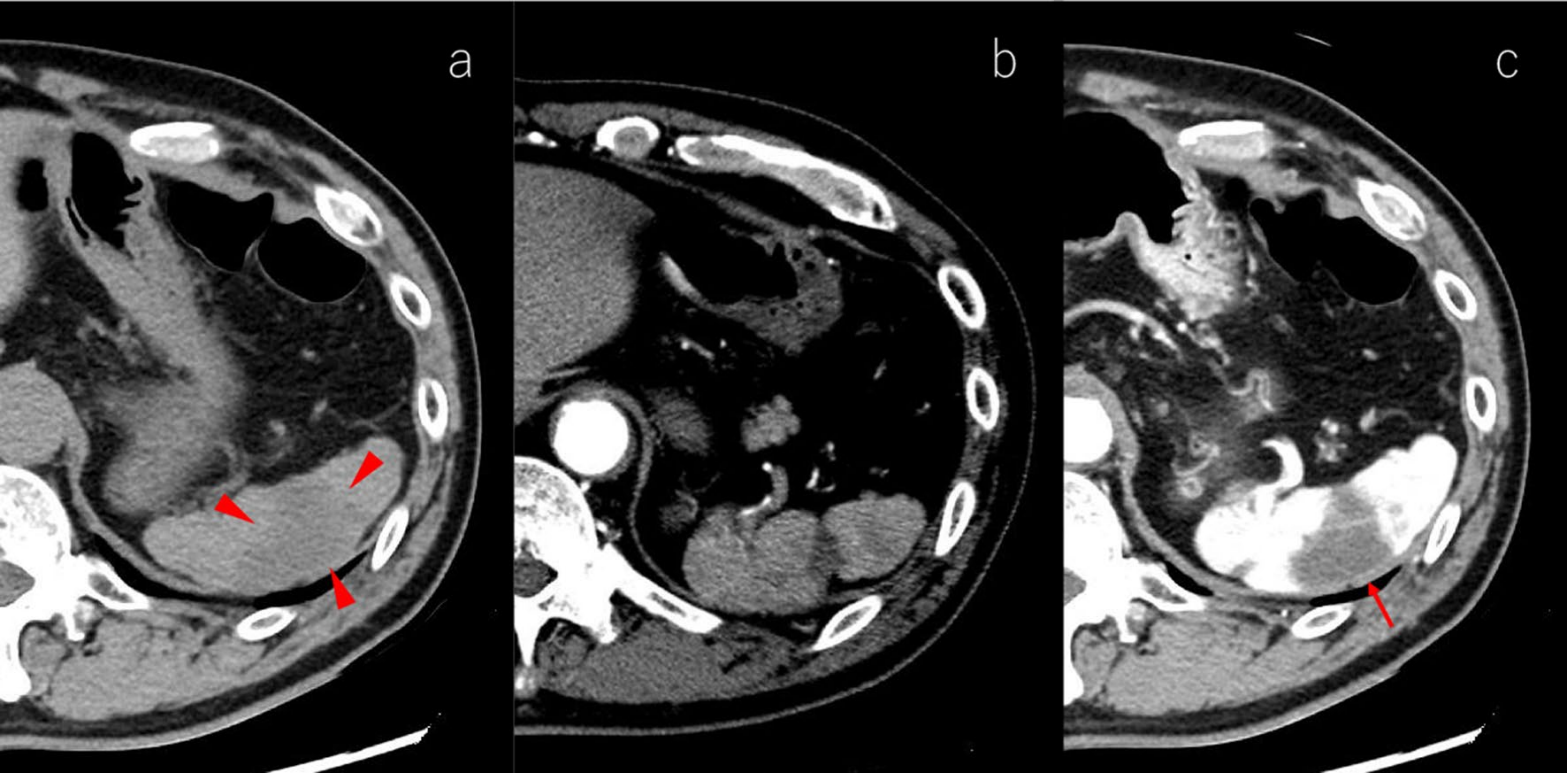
Plane and Enhanced CT findings (axial). a Plane CT shows a slightly low-density area (red arrowheads) compared with the surrounding area in the spleen. b, c Arterial and venous phases of enhanced CT indicate a poorly contrasted area in the spleen (red arrow)
